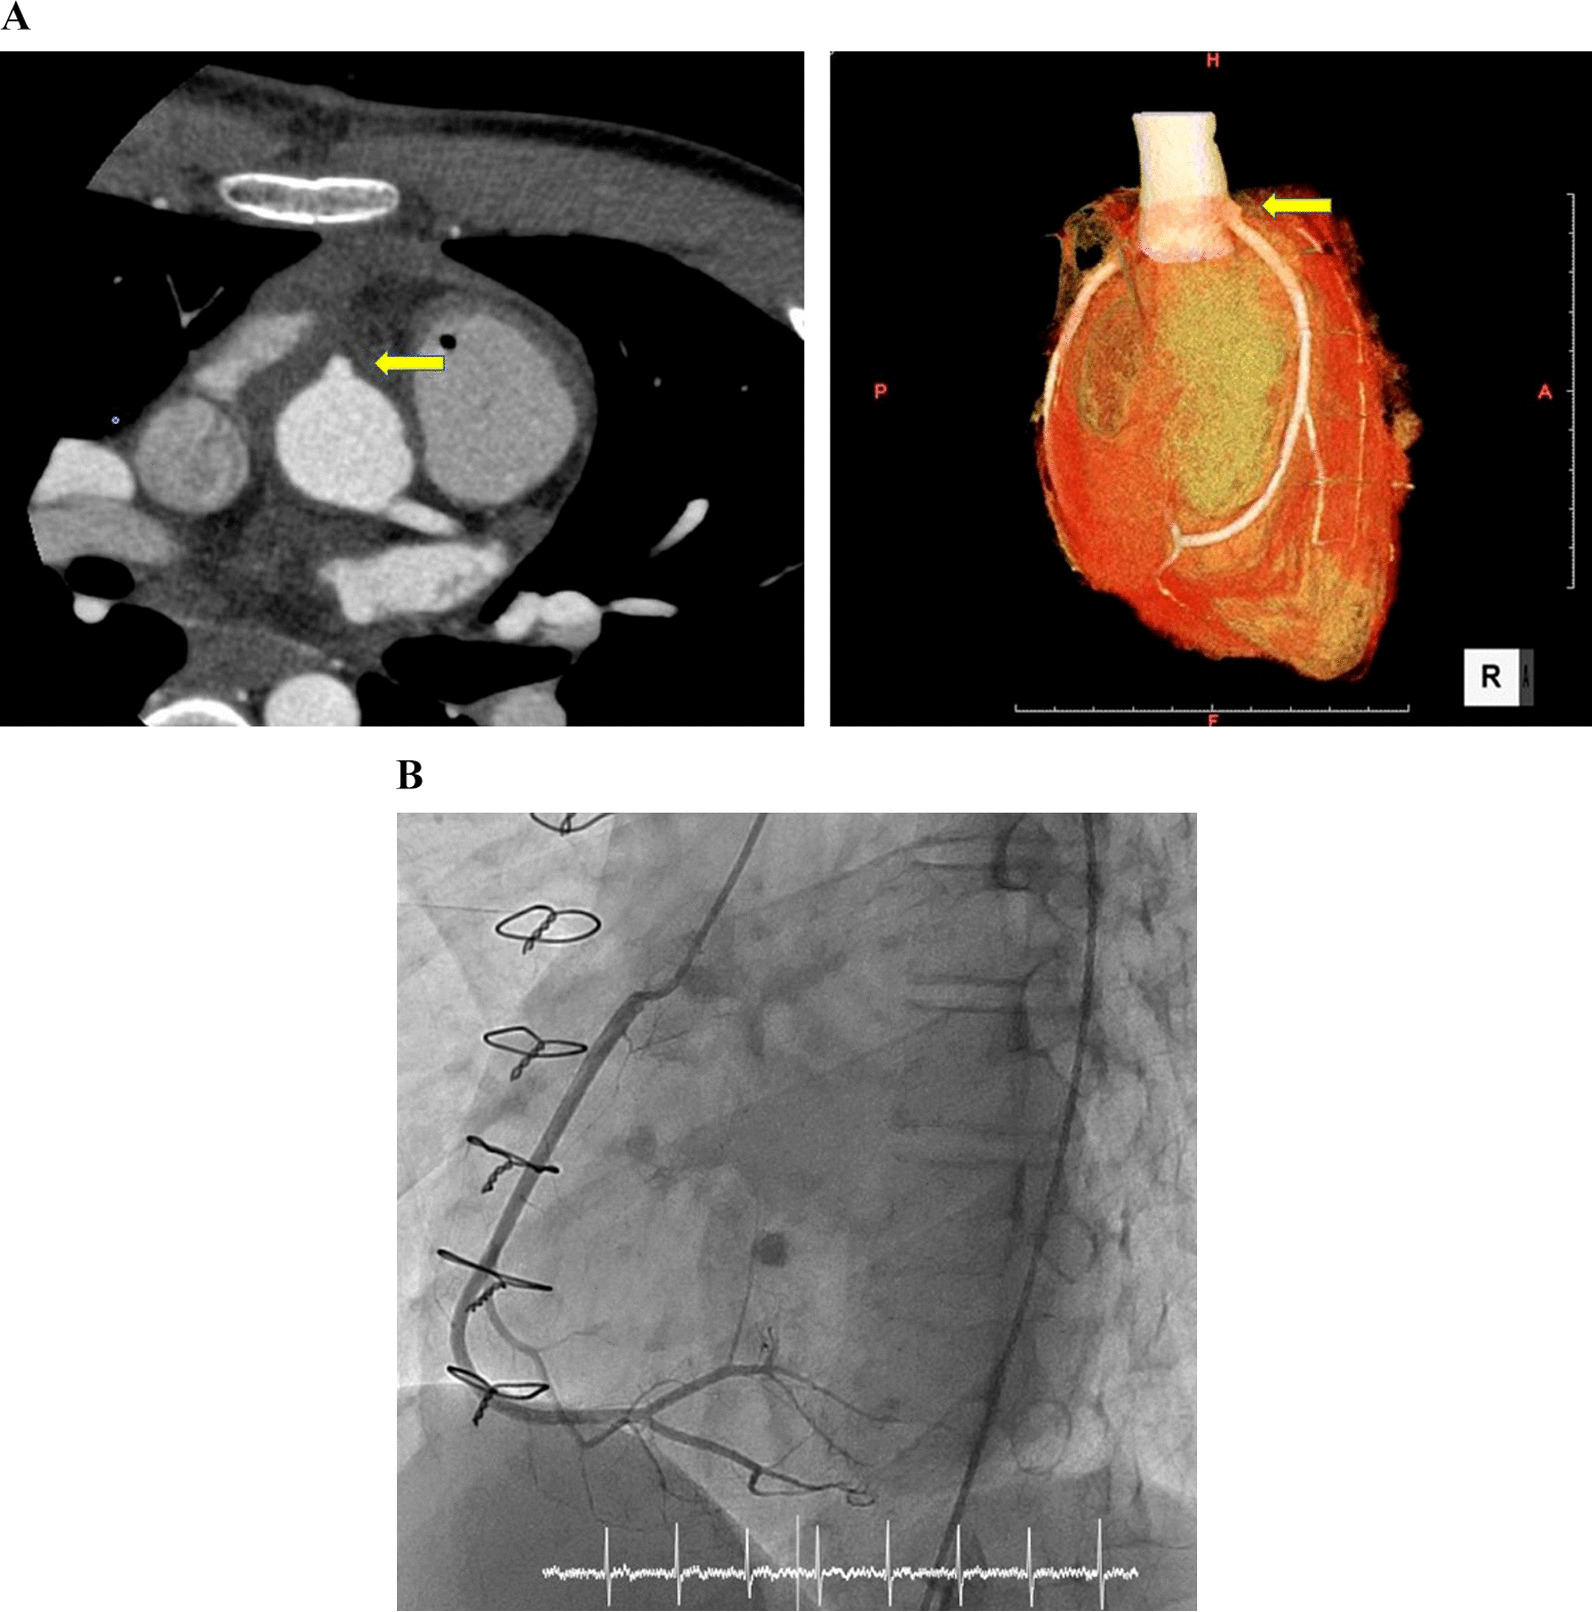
A Postoperative Coronary CT (computed tomography) revealed that anastomosis of the right coronary artery to the aorta was good. (Arrows indicate the anastomosis site. Right: axial image, Left: 3-D image). B Postoperative coronary angiography 3 month after operation
